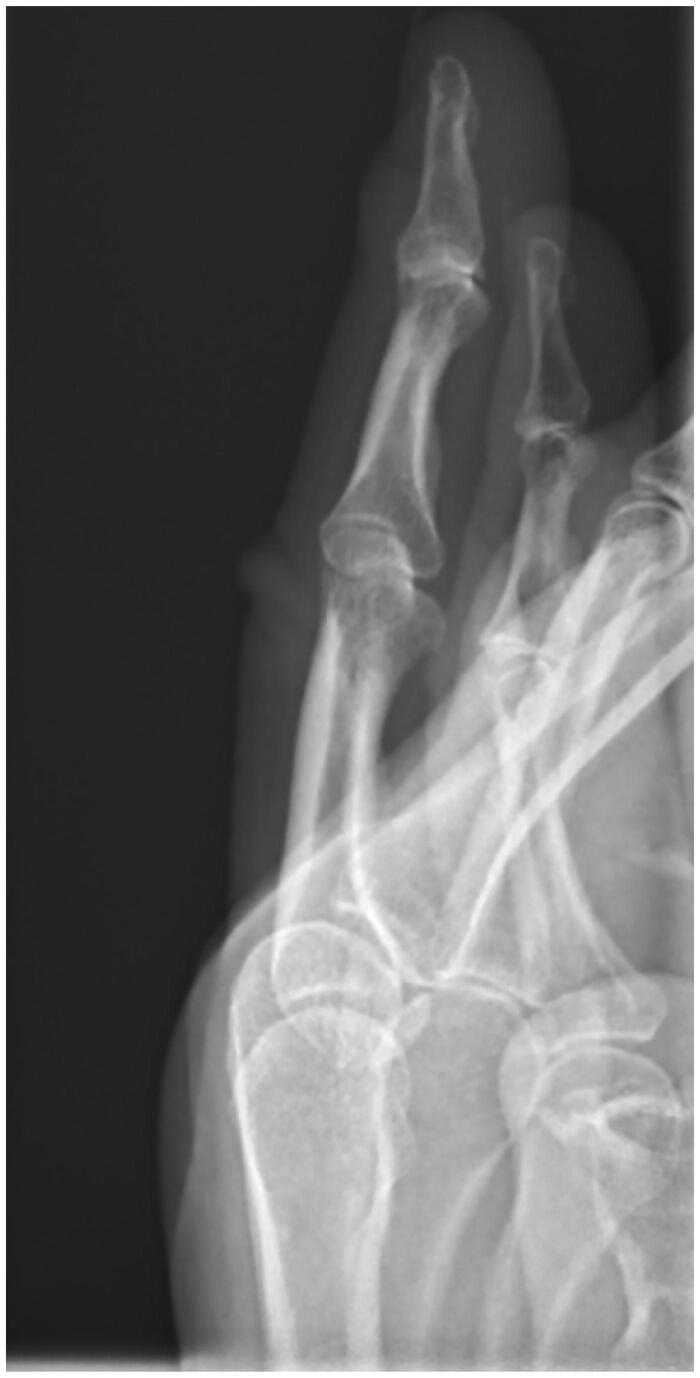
Lateral radiograph of the left ring finger.
